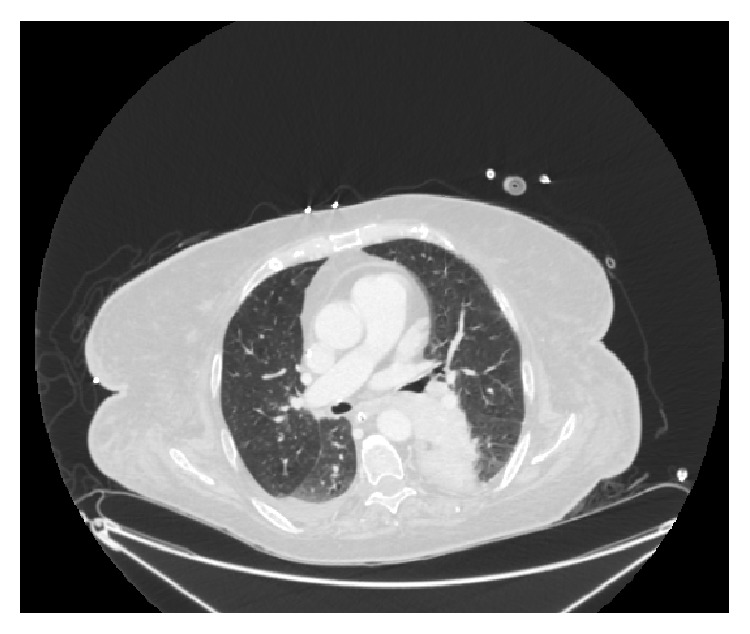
Computed tomography of the chest showing a large mediastinal obstructive mass in the left chest.
